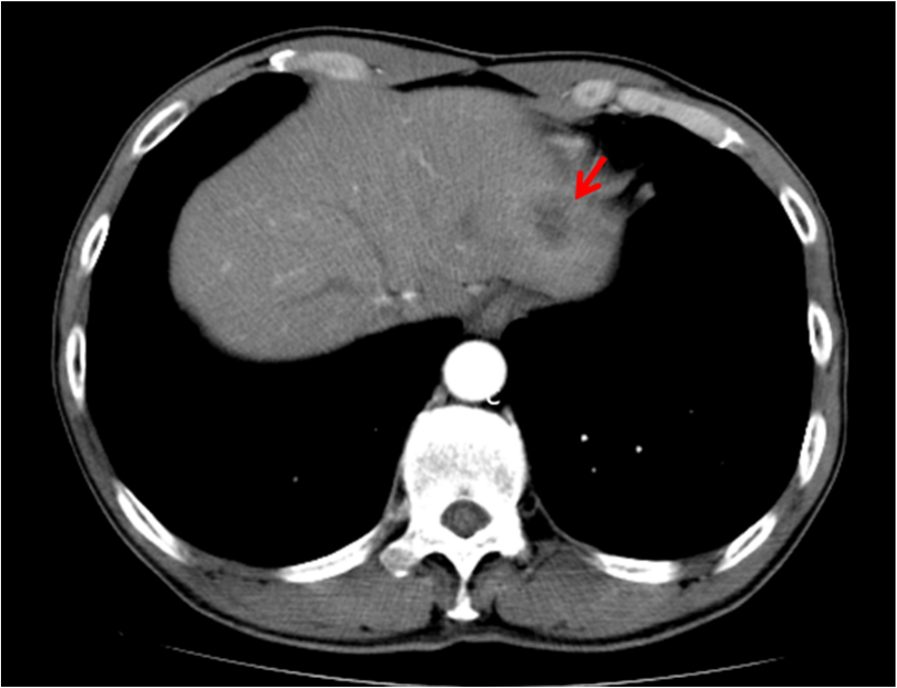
CT scan shows a hypovascularized tumor, measuring 30 mm, in hepatic S2 (arrow)
